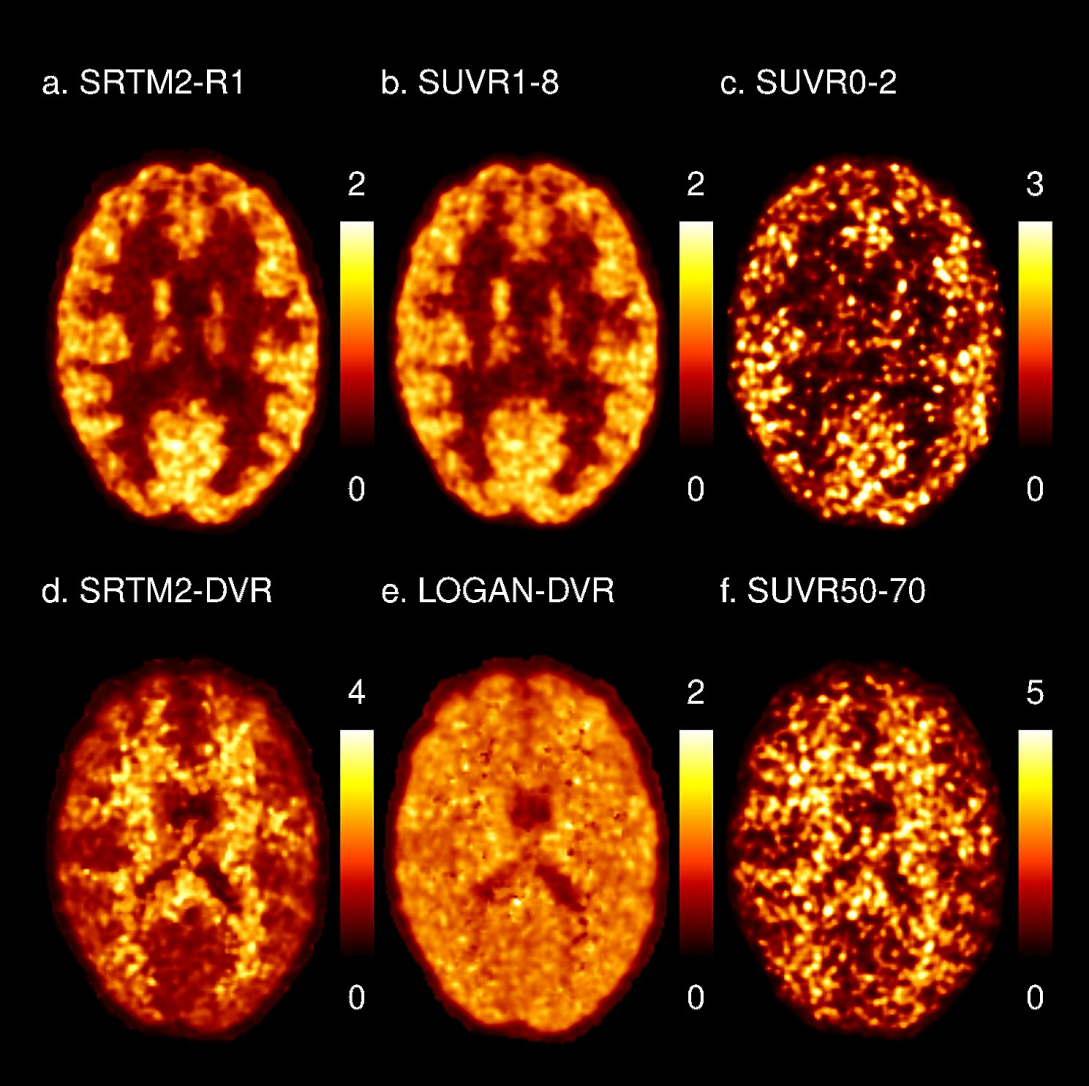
Parametric maps of perfusion and myelin content for a representative subject. The top row represents perfusion and the bottom row binding estimations. (A) SRTM2-R1: relative delivery of [^11^C]PiB as estimated by the SRTM2, (B) SUVR1-8: SUVR calculated on frames from 1 to 8 min, (C) SUVR0-2: SUVR calculated on frames from 0 to 2 min, (D) DVR-SRTM2: DVR as estimated by the SRTM2, (E) LOGAN-DVR: DVR as estimated by the Logan graphical reference method, (F) SUVR50-70: SUVR calculated on frames from 50 to 70 min
